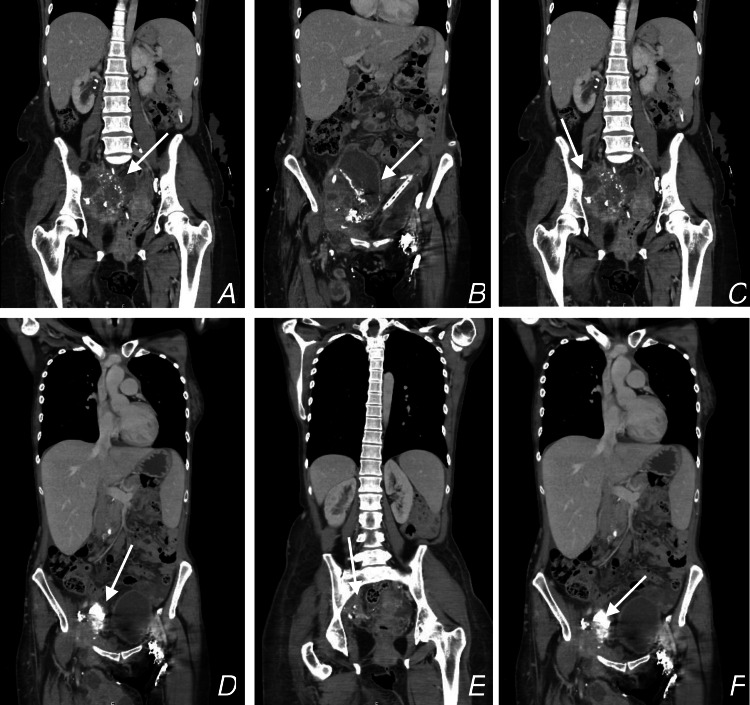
CT CAP images after debulking surgery A-E: Multiple coronal cuts of the CT CAP show the difference in tumor size before and after the debulking surgery. Arrows show an interval decrease in the size of pelvic sidewall tissue mass and an interval decrease in the size of right-inguinal metastatic lymph node with an increase in the size of areas of necrosis.
